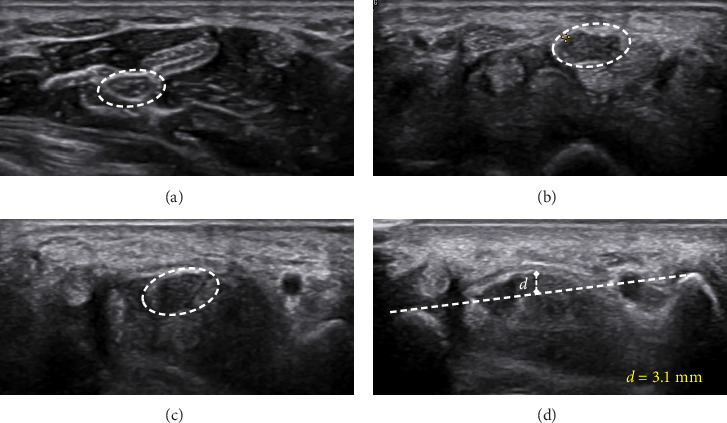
Transverse ultrasound of the right median nerve of a female with moderate CTS. (a) The CSAp is 0.06 cm^2^. (b) CSA at inlet of the carpal tunnel is 0.13 cm^2^. (c) CSA at outlet is 0.11 cm^2^. (d) The bowing of flexor retinaculum inlet is 3.1 mm.
